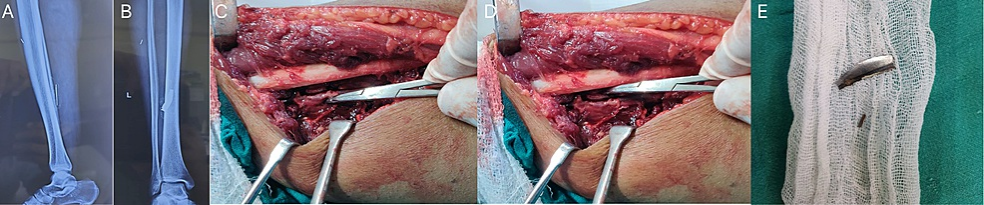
Radiographs, preoperative images, and extracted foreign body A 44-year-old male with a metal foreign body present in the posterolateral aspect of the tibia depicted radiographically (1A and B). Preoperative images (1C and D) using the posterolateral approach show close proximity of the foreign body (marked by haemostat in 1D) to the posterior tibial vessels as pointed by haemostat in figure 1C. Following extraction, the foreign body is seen to be a sharp steel projectile of size 3x1 cm (1E)
